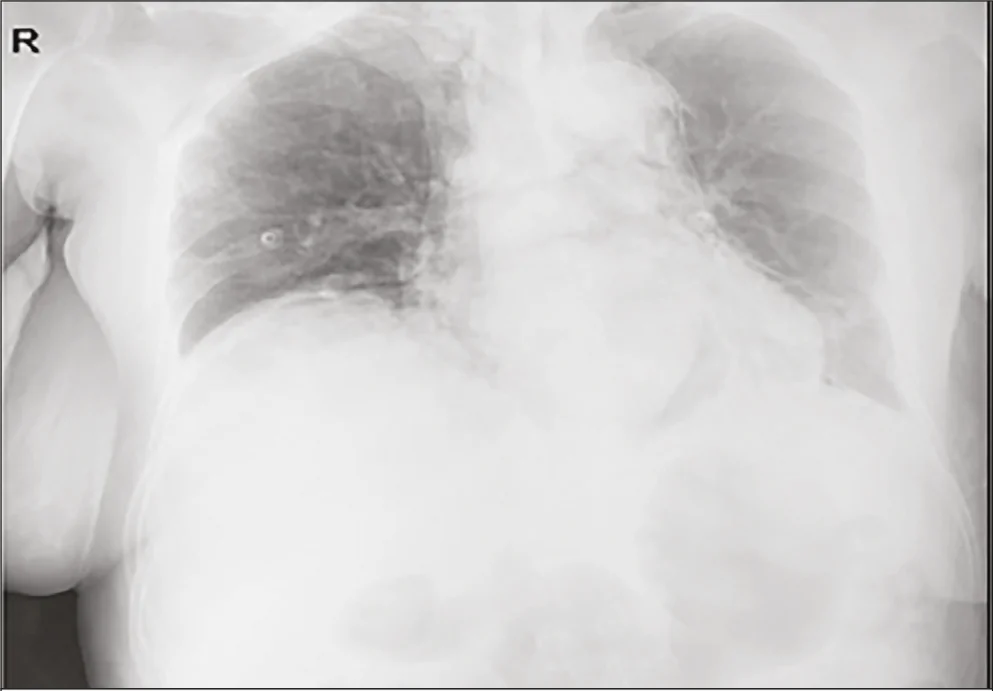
Chest X-ray after successful use of therapeutic plasma exchange.
